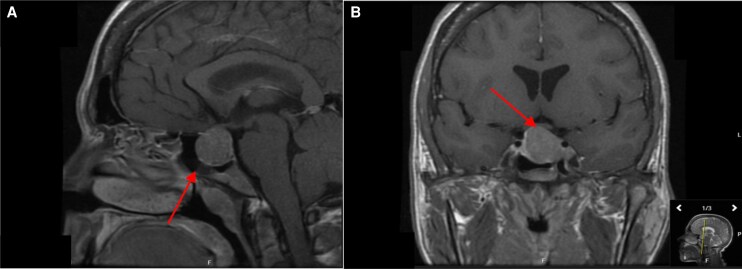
Postcontrast T1 magnetic resonance imaging demonstrating a homogenously enhancing sellar mass measuring 2.2 × 2.7 × 2.0 cm in the midsagittal (A) and coronal (B) planes.
